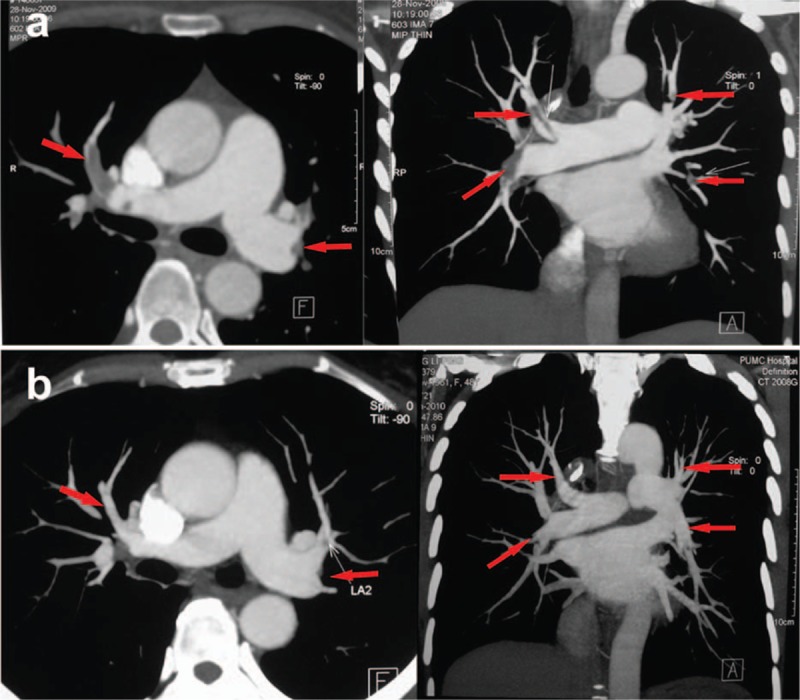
Change of the volume of thromboembolism before and after anticoagulation in case 1. (A) CTPA revealed multiple PTE in the pulmonary arteries of the left anterior superior lobe, left anterior inferior-medial lobe, right medial-middle lobe, and right inferior lobe. (B) CTPA showed that the PTE in the left anterior superior lobe, left anterior inferior-medial lobe, right medial-middle lobe, and right inferior lobe was dissolved. CTPA = computed tomographic pulmonary angiography, PTE = pulmonary thromboembolism.
